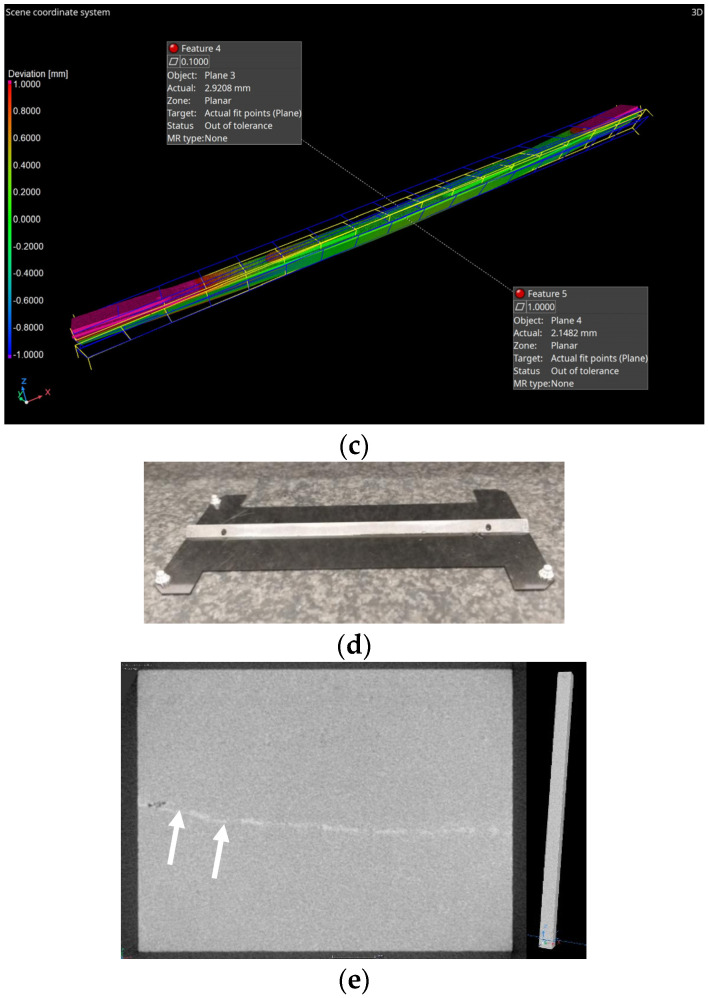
(a) General view showing severe bending and (b) CT cross-section of sample No.25 stored in extreme conditions. Flaws (identified by arrows) emerge in the glue volume. (c) The out-of-flatness is 2.9 mm while the lateral deflection is 2.15 mm. (d) General view of a subassembly including a GPI adapter. (e) CT cross-sectional view of the GPI adapter (sample No.7), also exposed to extreme conditions. Segregation bands and cavities (identified by arrows) are present in the bulk of the adapter, but there is no deformation of the sample.
